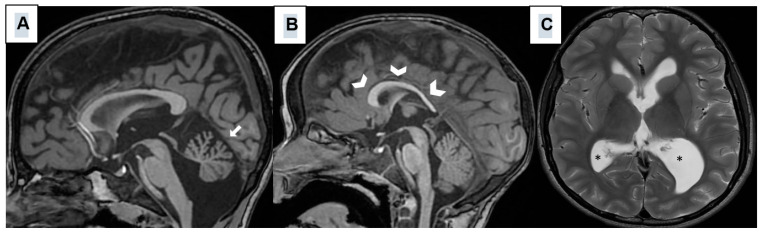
Sagittal T1-weighted (A,B) and axial T2-weighted (C) MRI images obtained from three different SHMS patients and showing hypoplasia/atrophy of the cerebellar vermis (white arrow); (A), thin and dysmorphic corpus callosum (thick arrowhead) (B), enlarged and asymmetric lateral ventricles (*) (C).
